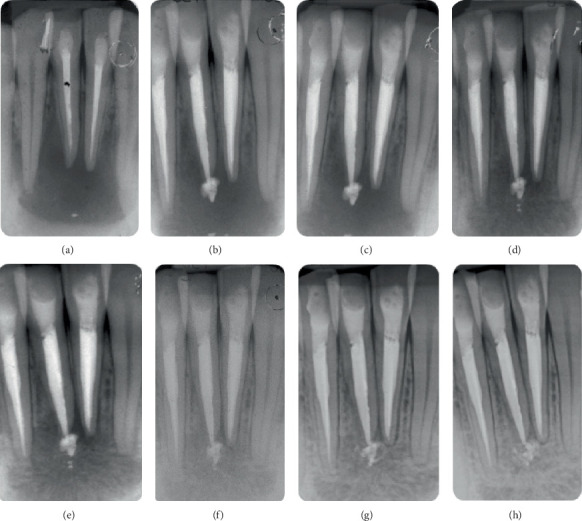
Timeline of radiographic evaluation of the periapical healing process. (a) Preoperative x-ray showing a huge periapical lesion involving Teeth 42, 41, and 31 with apical bone loss. (b) X-ray immediately after the endodontic treatments. Please note the periapical extrusion of the bioceramic sealer from Tooth 41. (c) X-ray at 3 months. The extrusion of the bioceramic sealer appears stable beyond the apex. (d) X-ray at 6 months showing initial bone healing and continued and stable presence of extruded sealer. (e) Radiograph at 12 months showing an improvement in bone regeneration and the initial integration of the extruded sealer into surrounding tissues. (f) Radiograph at 18 months with further signs of healing and no adverse reaction to the extruded sealer. (g) Radiograph at 24 months showing continued bone regeneration. (h) Radiograph at 30 months confirming complete resolution of the lesion, with almost full integration of the extruded sealer.
